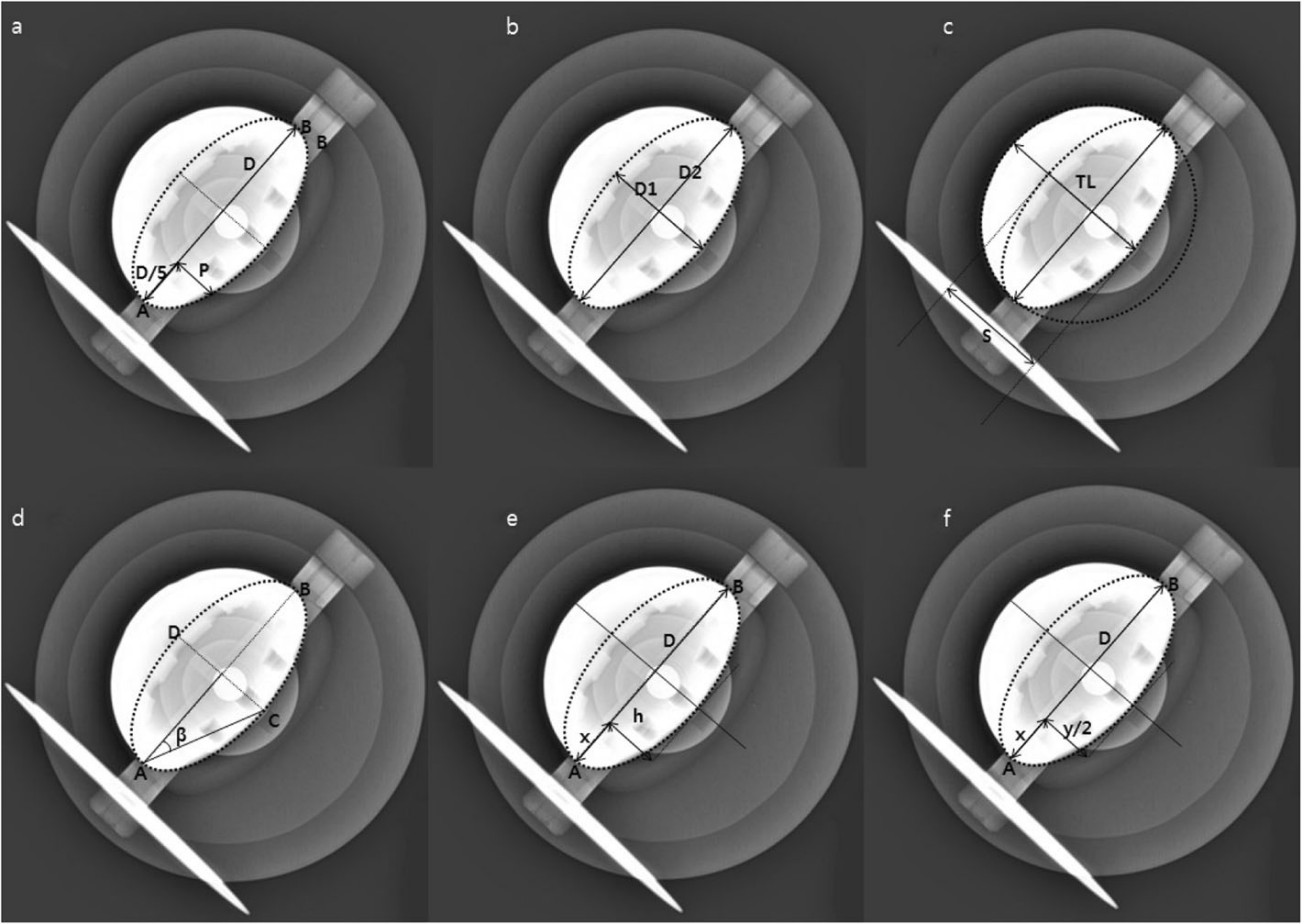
Methods for measuring anteversion on plane anteroposterior radiographs. a Pradhan et al. (arcsin (p/0.4D)), b Lewinnek et al. (arcsin (D1/D2)), c Widmer et al. (arcsin([S]/[TL]), d Liaw et al. (sin-1 tan β), e Hassan et al. ([arcsin [(h/D) / √([m/D] – [m2/D2])]], f Ackland et al. (arcsin [2y / 2√(2Dx – x2)])
